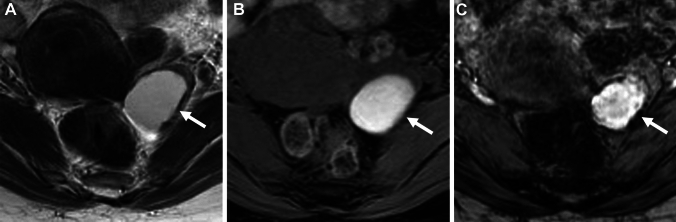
Endometrioma. A 45-year-old with a history of dysmenorrhea and suspicion of a left ovarian cyst on ultrasonography examination. A T2-weighted image reveals a high signal intensity left ovarian cyst (arrow). B On fat-saturated T1-weighted image, the left ovarian cyst (arrow) shows high signal intensity. C SWAN reveals dotty to curved linear signal voids in the cyst wall (arrow), which suggest endometrioma
